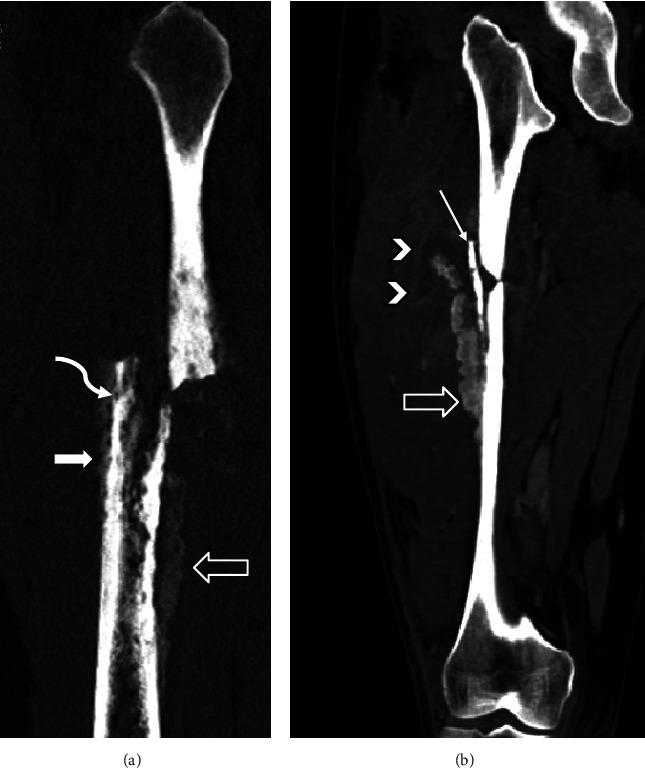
CT scan of right femur in the same patient 3 days after fracture. (a) Sagittal reformat of noncontrast CT image shows both organized (open arrow) and disorganized periosteal reactions (white arrow). The permeative bone destruction is best depicted on CT (curved arrow). (b) Coronal reformat of contrast CT image shows a low-density collection/lesion with a thin enhancing rim (arrowhead) around the fracture. The organized thick periosteal bone formation (open arrow) surrounds a very dense spicule that resembles necrotic bone or sequestrum (long thin arrow).
